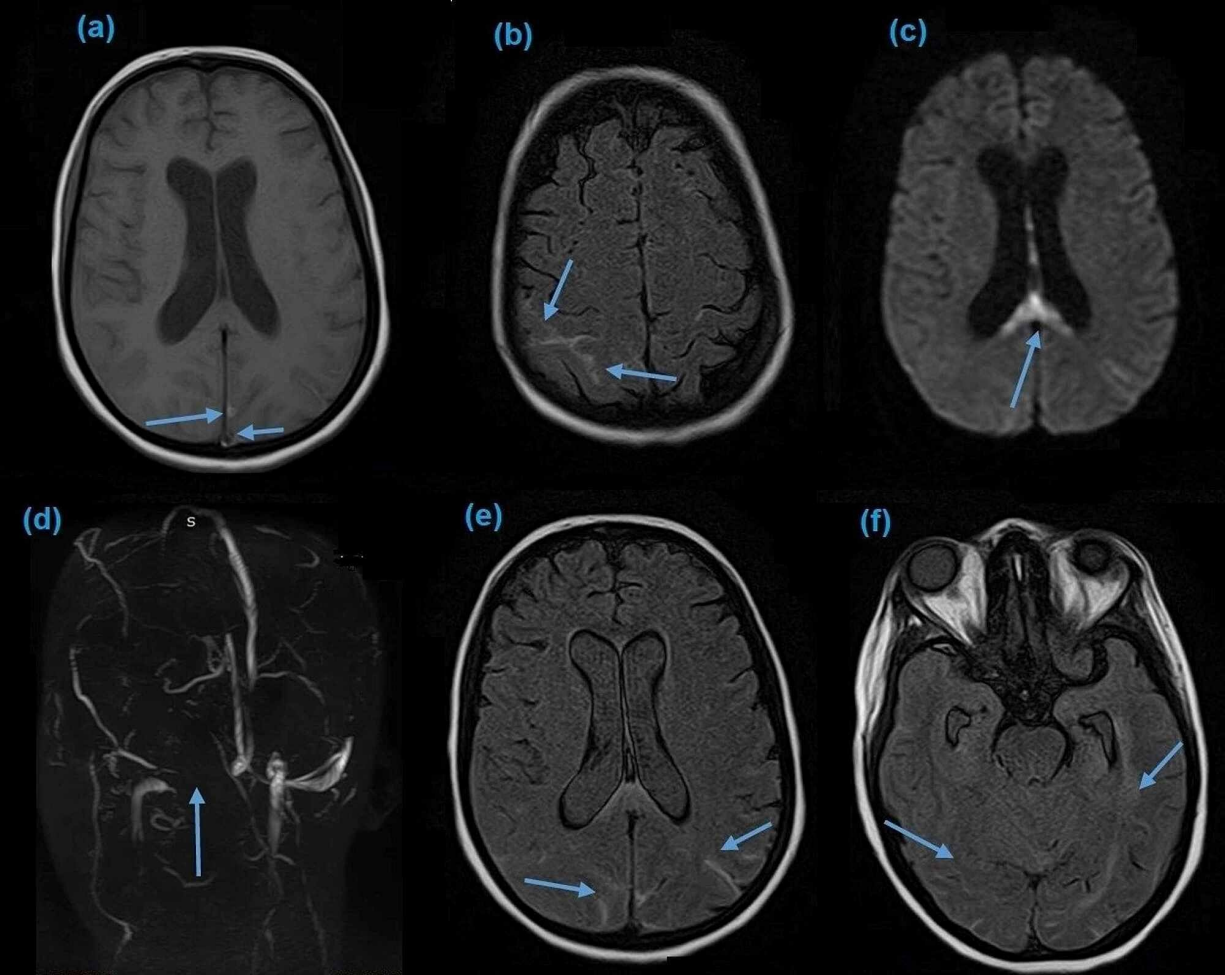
Axial images of the brain MRI showing DVST and SAH; high signal linear intensities in T1-weighted image, more prominent in the parietal lobe (a and b), diffusion restriction in the splenium of corpus callosum (c), loss of visualization of right sinus transverse on MRV (d), hyperintensity in bilateral sulci of the cortex (e), and hyperdense material filling the subarachnoid space (f). MRI: magnetic resonance imaging; DVST: dural venous sinus thrombosis, SAH: subarachnoid hemorrhage, MRV: magnetic resonance venography
